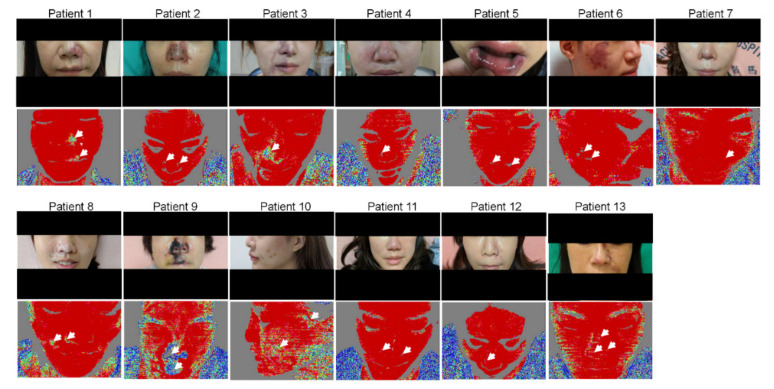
Clinical photographs and laser Doppler imaging of all patients. At Day 1 of admission, the clinical photographs and DPI represent arterial occlusions in the region of facial filler injection (closed arrows).
